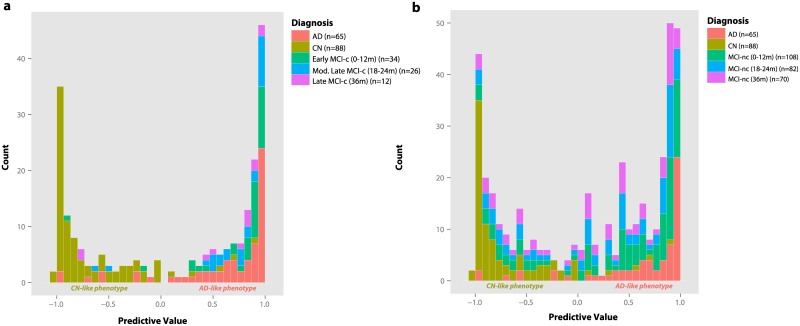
Predictive values from the combined CSF RFE subset CSF biomarker and regional MRI measures model for MCI to AD conversion prediction at several follow up timepoints. (A) Predictive values of MCI-c progressing to AD at different follow up timepoints overlaid with predictive values of AD and CN individuals and (B) predictive values of MCI-nc at different follow up timepoints overlaid with predictive values of AD and CN individuals.
